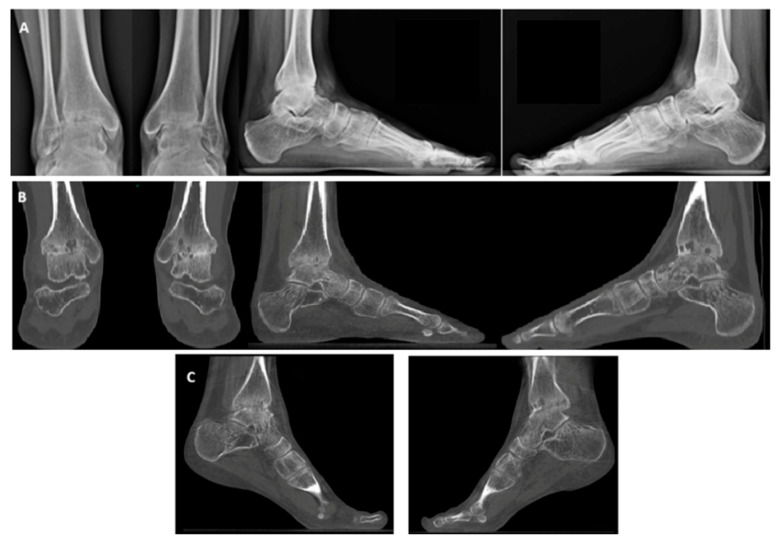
Hemophiliac patient suffering from bilateral ankle osteoarthritis. Preoperative images: X-rays (A), weight-bearing CT, AP and side view (B), and plantar flexion weight-bearing CT (C).
